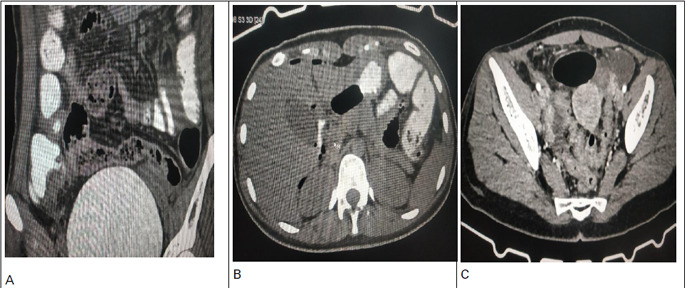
A) CT scan abdomen and pelvis showed thickening with ulcerated areas and subtle wall defect in the medial wall of the cecum with oral contrast extravasation, B) Pneumoperitoneum and C) Mild to moderate peritoneal collection.
